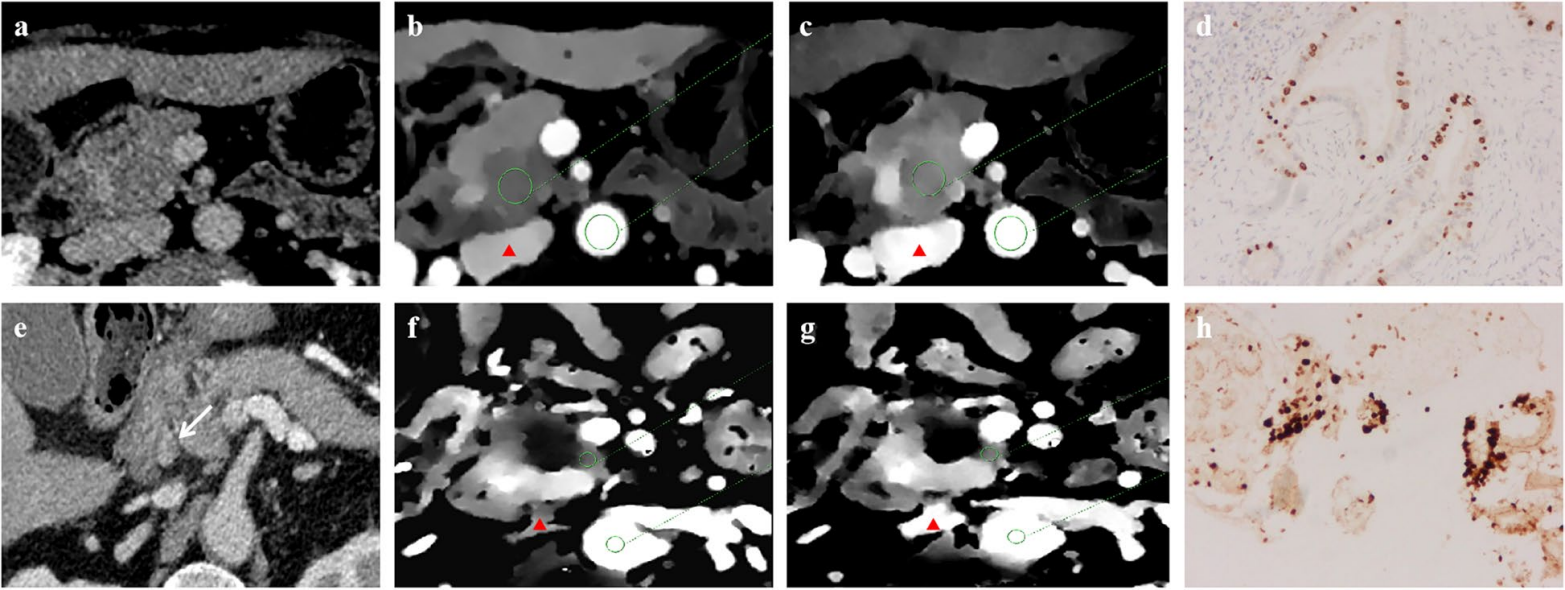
DECT figures of a 66-year-old female patient with low Ki-67 expression in PDAC. a CT-reported regional LN negative. b, c IC_tumour_ and IC_aorta_ in the same layer in the PVP and EP, the NIC of PVP was 0.45 mg/ml and the ECVf was 42.29%. d Ki-67 was 30%. (red arrow: inferior vena cava). DECT figures of a 73-year-old male patient with high Ki-67 expression in PDAC. e CT-reported regional LN positive. f, g IC_tumour_ and IC_aorta_ in the same layer in the PVP and EP, the NIC of PVP was 0.34 mg/ml and the ECVf was 37.08%. h Ki-67 expression was 60%. (red arrow: inferior vena cava). DECT, dual-energy computed tomography; ECVf, extracellular volume fraction; EP, equilibrium phase; IC, iodine concentration; LN, lymph node; NIC, normalized iodine concentration; PDAC, pancreatic ductal adenocarcinoma; PVP, portal vein phase
